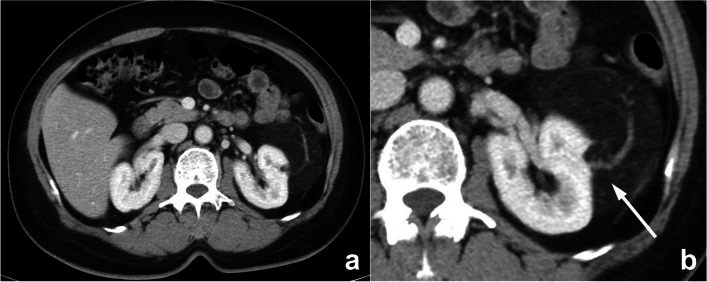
A mass revealed by physical examination in the left kidney of a 58-year-old woman. a An enhanced, axial abdominal CT image showed a left pararenal fat-density mass, which was diagnosed as liposarcoma. However, this lesion should have been diagnosed as angiomyolipoma instead of liposarcoma. The misdiagnosis may be due to missing key signs such as large blood vessels (arrow) in the tumor and the renal cortical “split sign”
